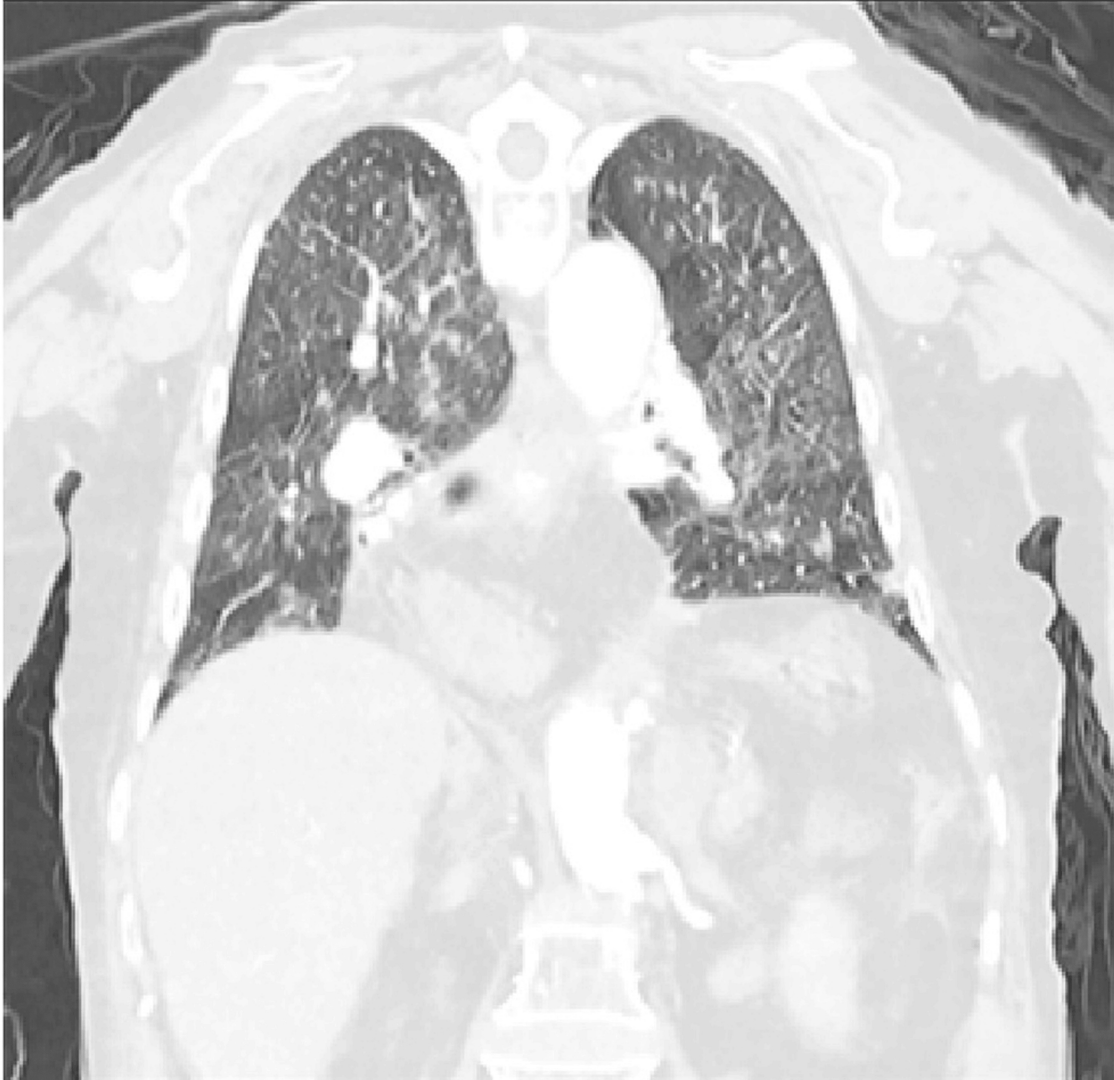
Coronal CT chest on day four of admission demonstrating widespread irregular, subcentimeter nodules with subtle ground glass peripheries suggestive of viral pneumonitis, but no frank consolidation.
